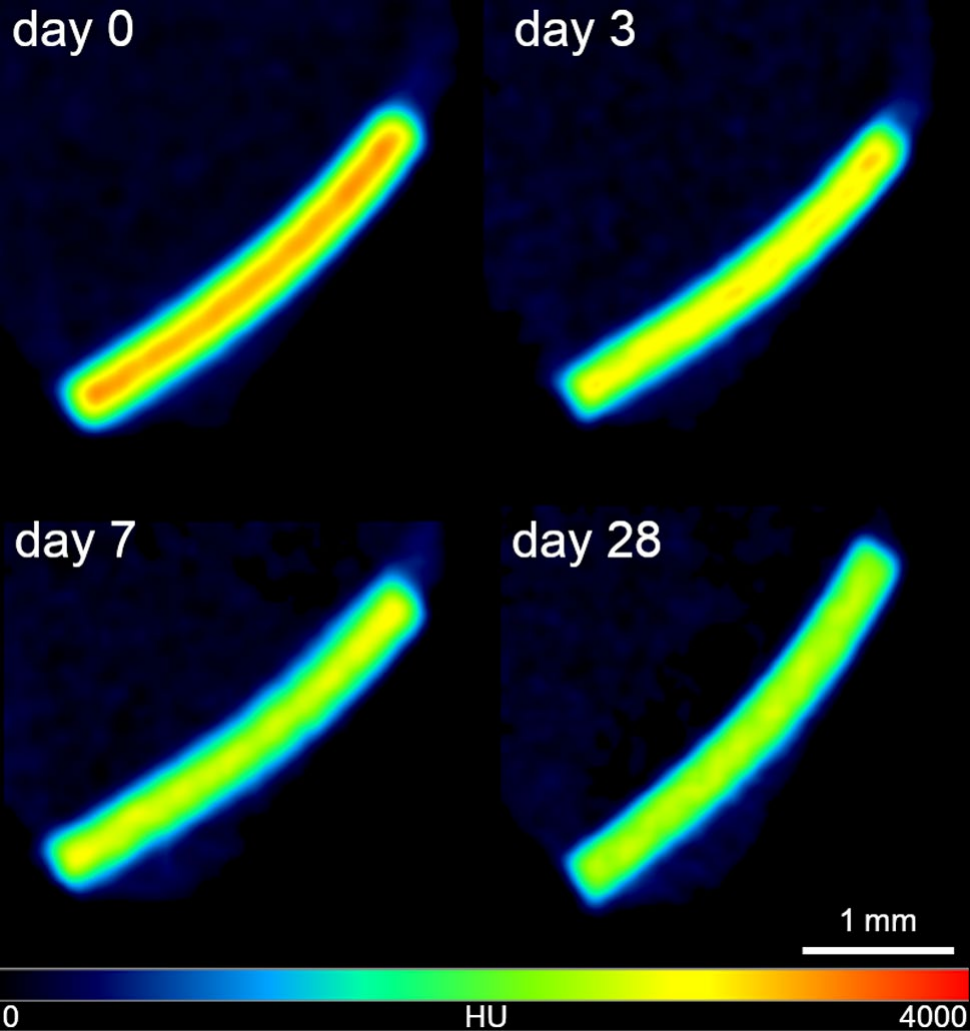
CT Image in hot/cold view of a 0.3 × 3 mm PLGA implant on day 0 and after incubation in PBS on days 3, 7, and 28
